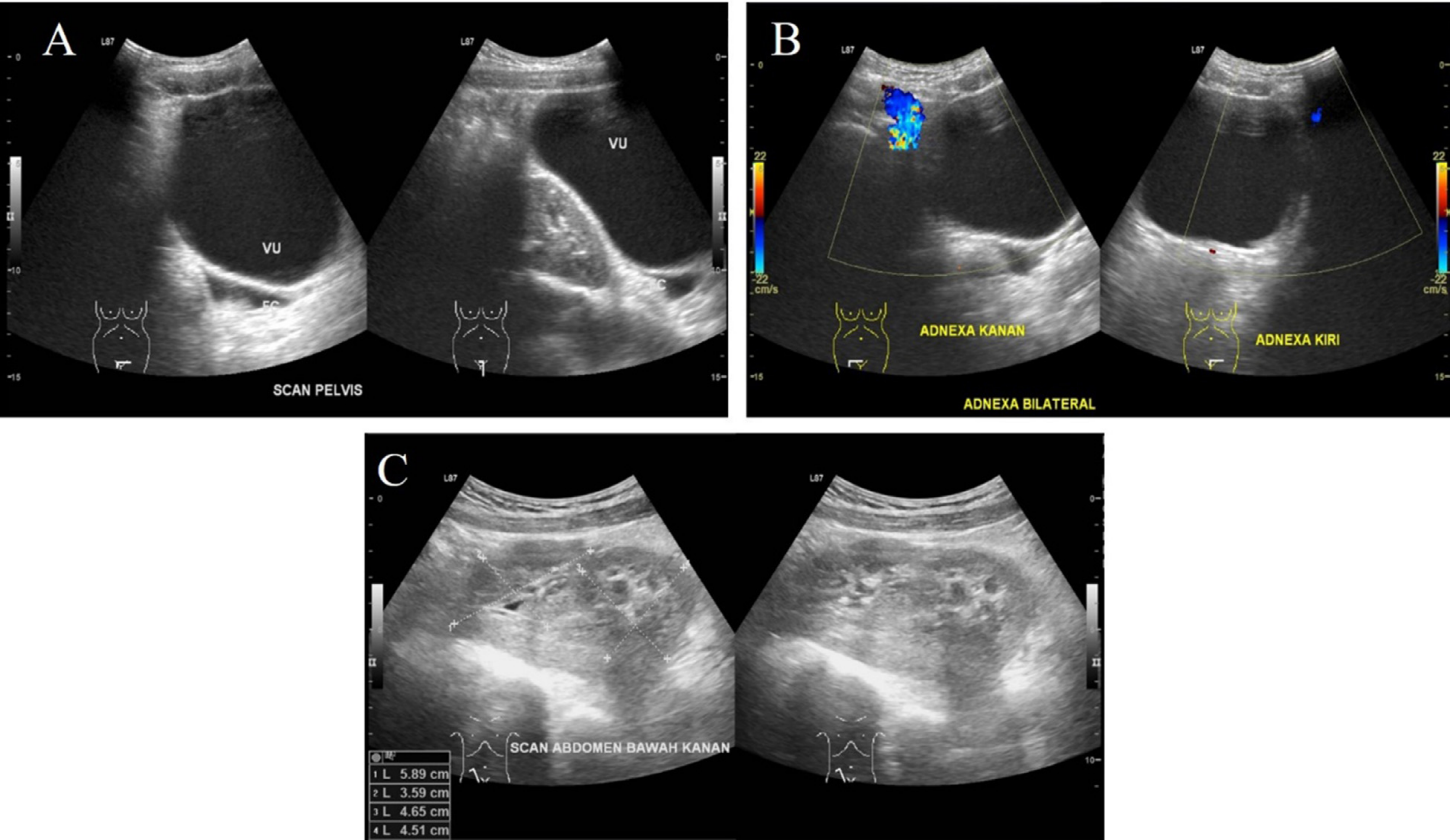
(A and B) Structure of normal uterus was not seen. (C) Lower abdominal ultrasound revealed ectopic kidney within the right pelvic cavity, ureter was difficult to be observed.
